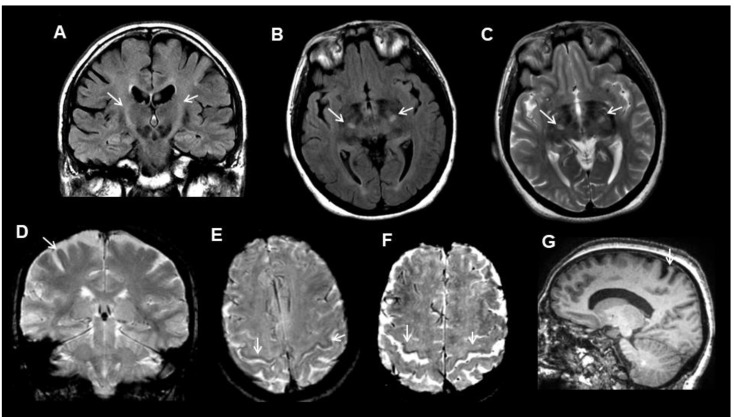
Examples of the MRI (Magnetic Resonance Imaging) features (arrows) in amyotrophic lateral sclerosis (ALS) patients. (A) corticospinal tract FLAIR hyperintensity in a coronal image. (B) corticospinal tract fluid attenuated inversion recovery (FLAIR) hyperintensity in an axial image. (C) corticospinal tract T2 hyperintensity in an axial image. (D) motor cortex T2* hypointensity in a coronal image. (E) motor cortex SWI hypointensity in an axial image. (F) motor cortex SWI hypointensity and widening of the central sulcus in an axial image. (G) selective motor cortex atrophy in a sagittal-T1 image.
